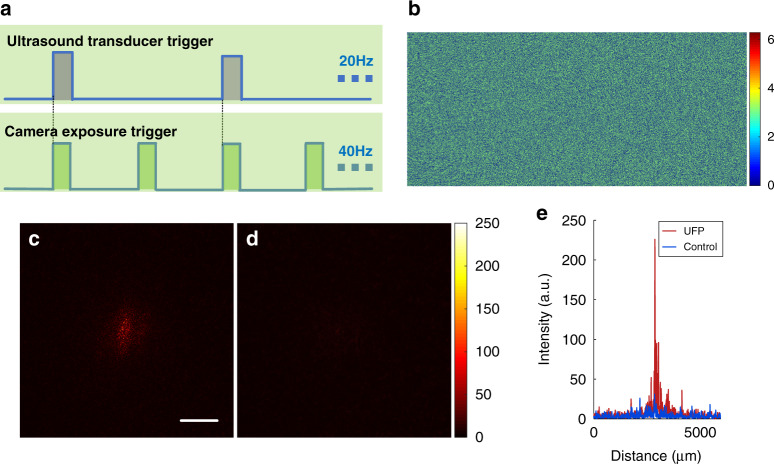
Double-shot UFP optical focusing into scattering media. a Time sequence for double-shot UFP optical focusing. b Phase map generated from the two recorded hologram frames. c Time-reversed focus from double-shot UFP optical focusing. d In a control experiment, no focus was observed on the observation camera in a double-shot measurement without ultrasound. e Line profiles of the central rows in c and d. Scale bar: 1 mm
